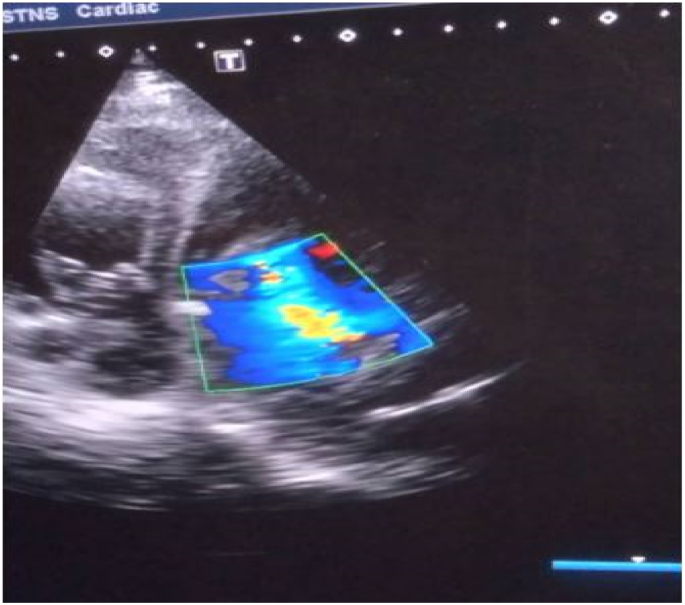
Transthoratic echocardiography shows moderate mitral regurgitation with posterior eccentric jet.
